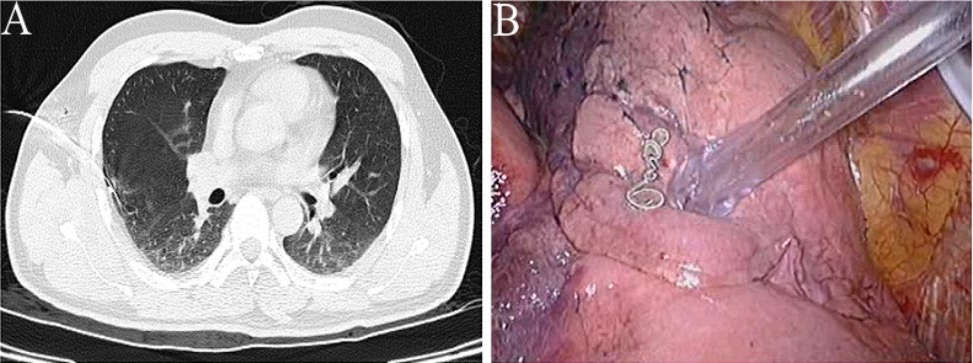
Images of lung CT scan and surgical field for the breakaway of marking materials. (A) A breakaway of the coil was observed after the trocar needle was withdrawn. (B) A breakaway coil was found in the pleural cavity during VATS
